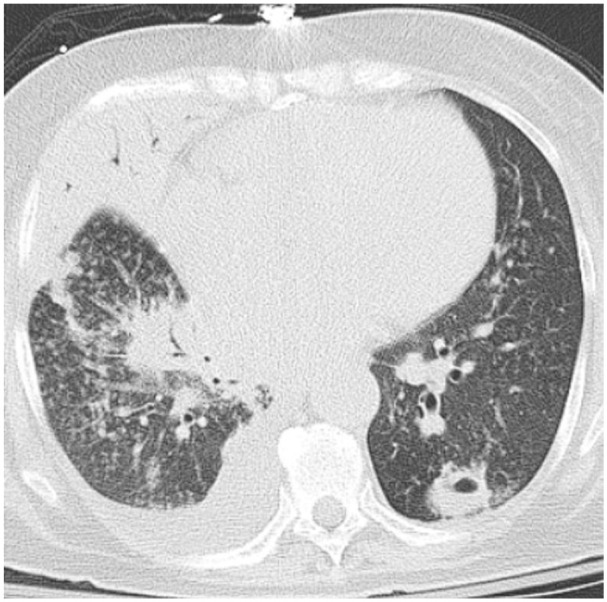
CT chest without contrast demonstrated right-sided pleural effusion with cavitary pulmonary lesions.
